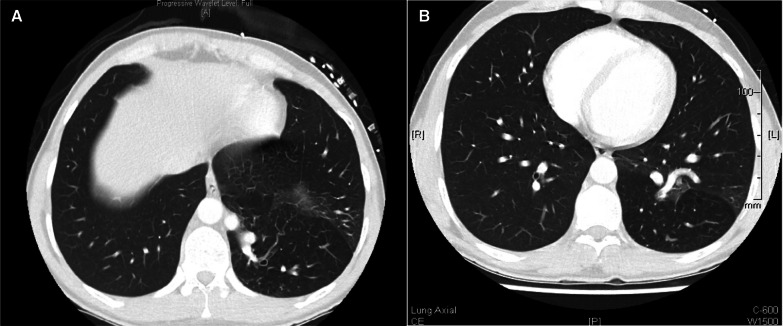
A, B CT scan showing aberrant formation of segmental lung tissue within the left lower lobe
